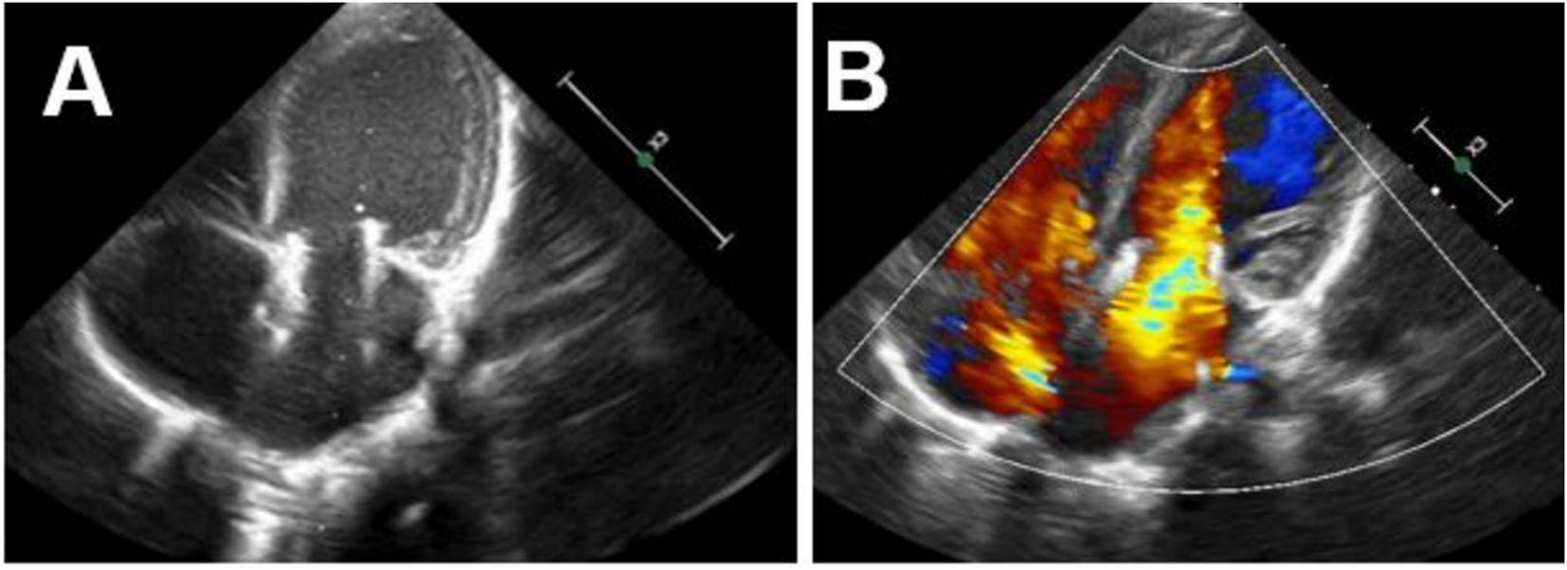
Follow-up echocardiography eight-month post-procedure. A Apical 4 C in systole with Melody valve with adequate opening. B Apical 4 C with color Doppler without evidence of significant flow acceleration, with a mean valvular gradient of 4.5 mmHg
